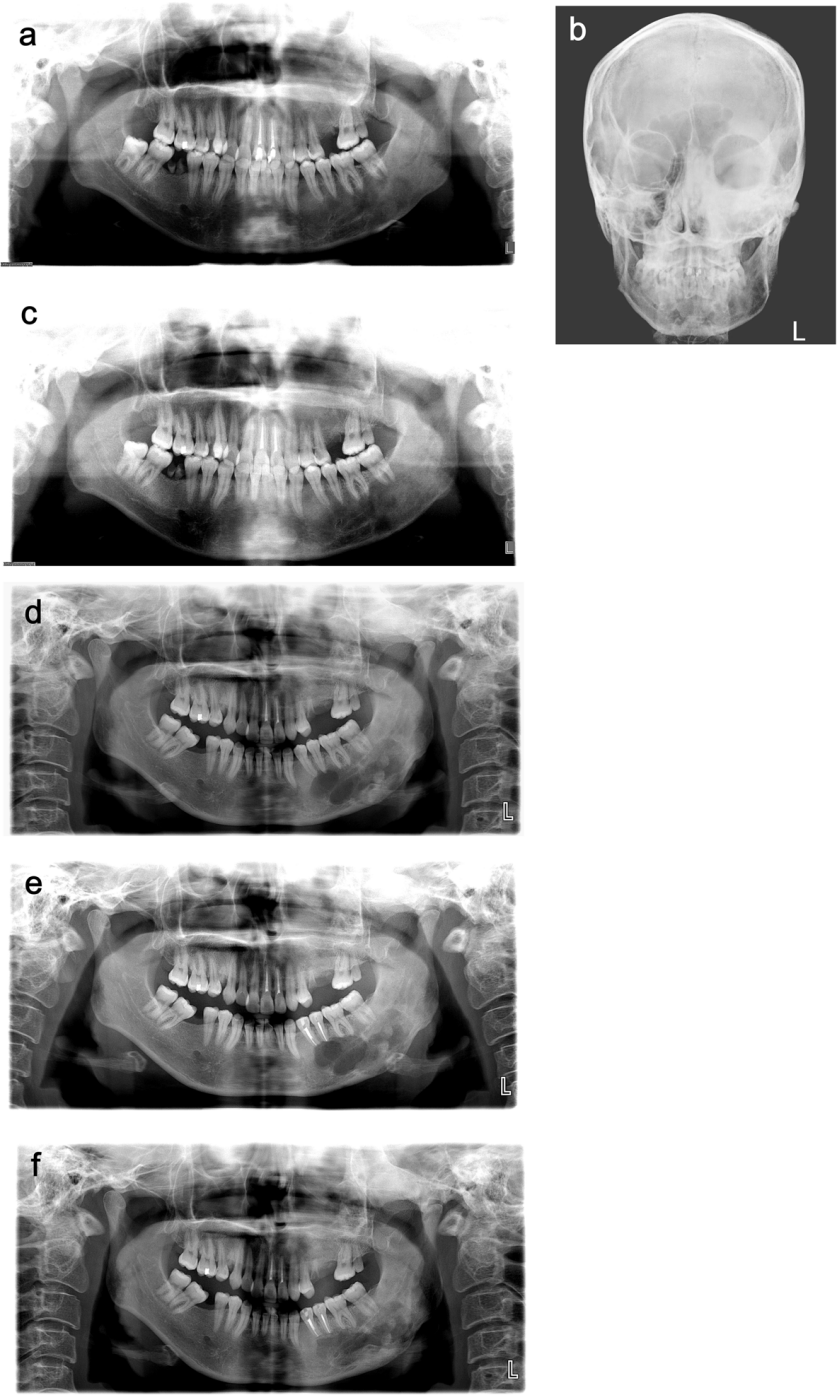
Radiographic images of the patient’s jaw and skull. a Panoramic view of the jaw at baseline taken at the first visit to the out-patient department (2007.5). b Posteroanterior projection (PA) view of the skull at baseline taken at the first visit to the out-patient department (2007.5). c Postoperative panoramic view of the jaw taken 18 months post-surgery (2008.11). d Panoramic view of the jaw taken at the out-patient department (2018.11). e Panoramic view of the jaw after cyst enucleation of the left mandibular lesion. f Postoperative panoramic view of the jaw 6 months after the surgery in November 2018 (2019.5)
